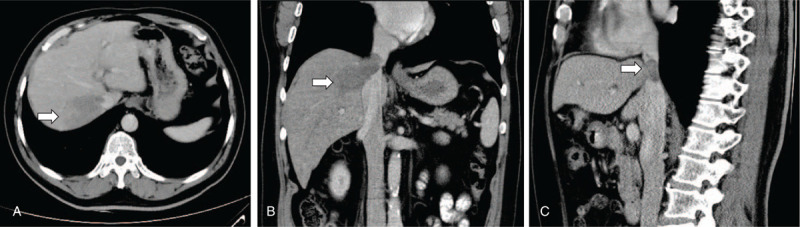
Dynamic contrast-enhanced CT scan findings. A: A hepatic mass approximately 4.3 cm × 6.3 cm in size in segment VII of the liver in the cross-section. B: A hepatic mass with thrombus in the IVC in the coronal plane. C: A thrombus in the IVC in the sagittal plane. IVC = inferior vena cava.
